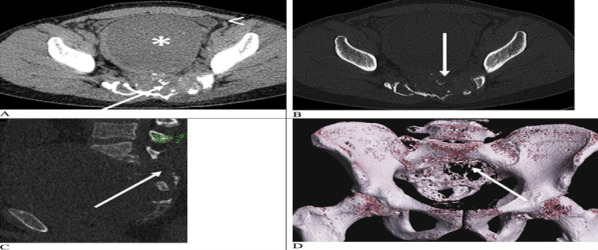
Axial soft tissue and bone window computed tomography of the pelvis (a and b) shows large lytic expansile midline lesion, destruction of sacrum with bilateral neural foraminal and sacral canal components. Multiple calcific foci are seen within the mass (white arrows). The lesion has large, predominantly cystic presacral component (* in a), which is seen displacing urinary bladder anteriorly (arrowhead in a). Sagittal bone window computed tomography (c) and volume-rendered images (d) shows lytic destruction of the sacrum (white arrows in c and d).
